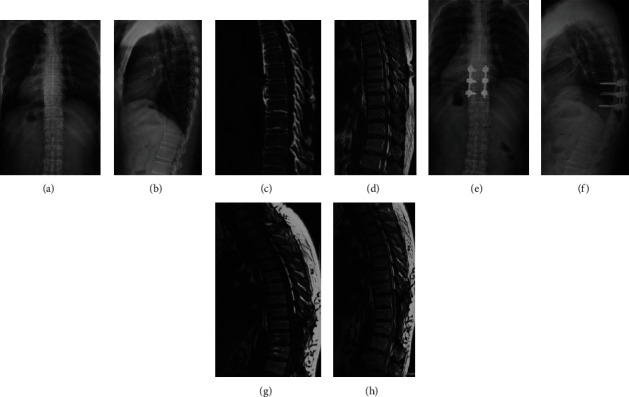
A 49-year-old patient with STOLF from T9 to T11 levels who received laminectomy. (a, b) Preoperative standing anteroposterior X-ray. (c) Preoperative CT. (d) Preoperative MRI. (e, f) Postoperative standing anteroposterior X-ray at final follow-up. (g, h) Postoperative MRI at final follow-up.
